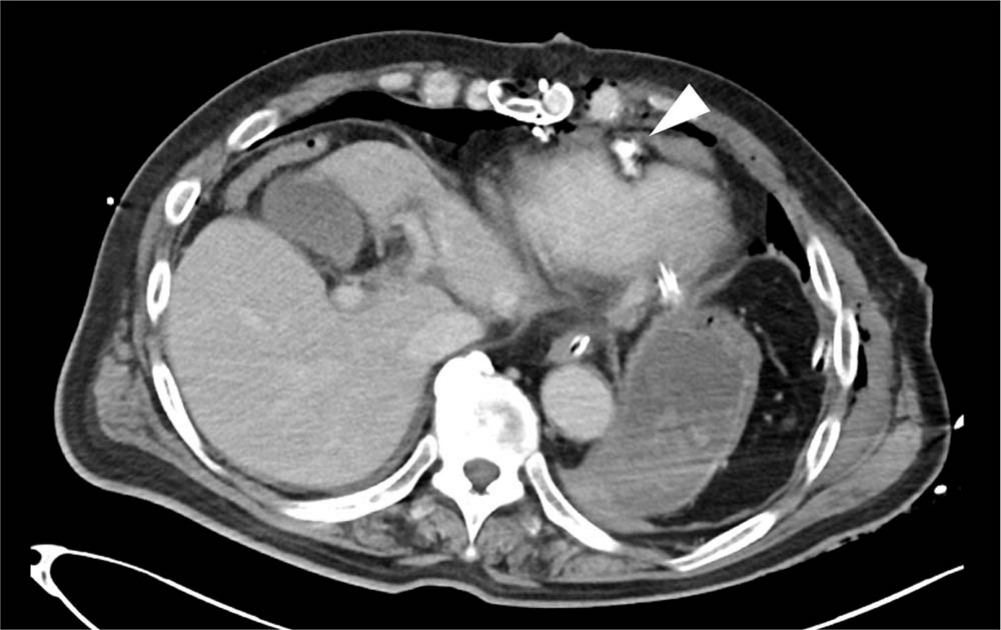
Postoperative CT findings. No evidence of pericardial effusion was observed, and the surgical site was hemostatic (arrowhead).
